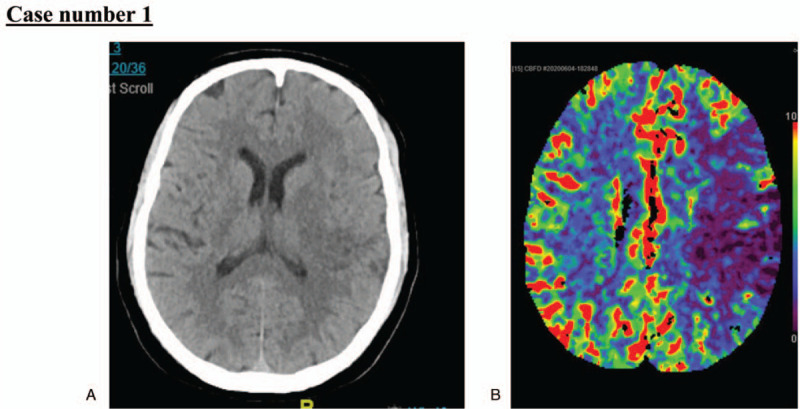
A. Unenhanced computed tomography and B. Brain perfusion scan revealing left middle cerebral artery (MCA) ischemic stroke.
